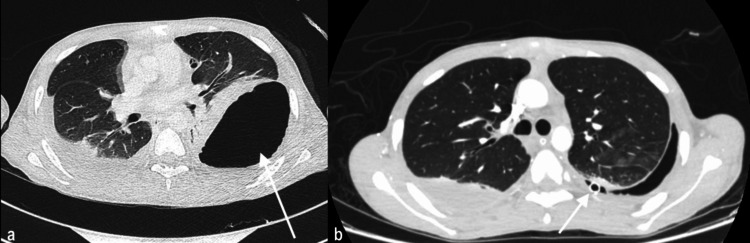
Follow-up CT scan (a). Development iatrogenic pneumothorax post malposition of ICT with bilateral pleural effusion. (b). Improvement of the pneumothorax with pleural effusion post reinsertion of ICT.
